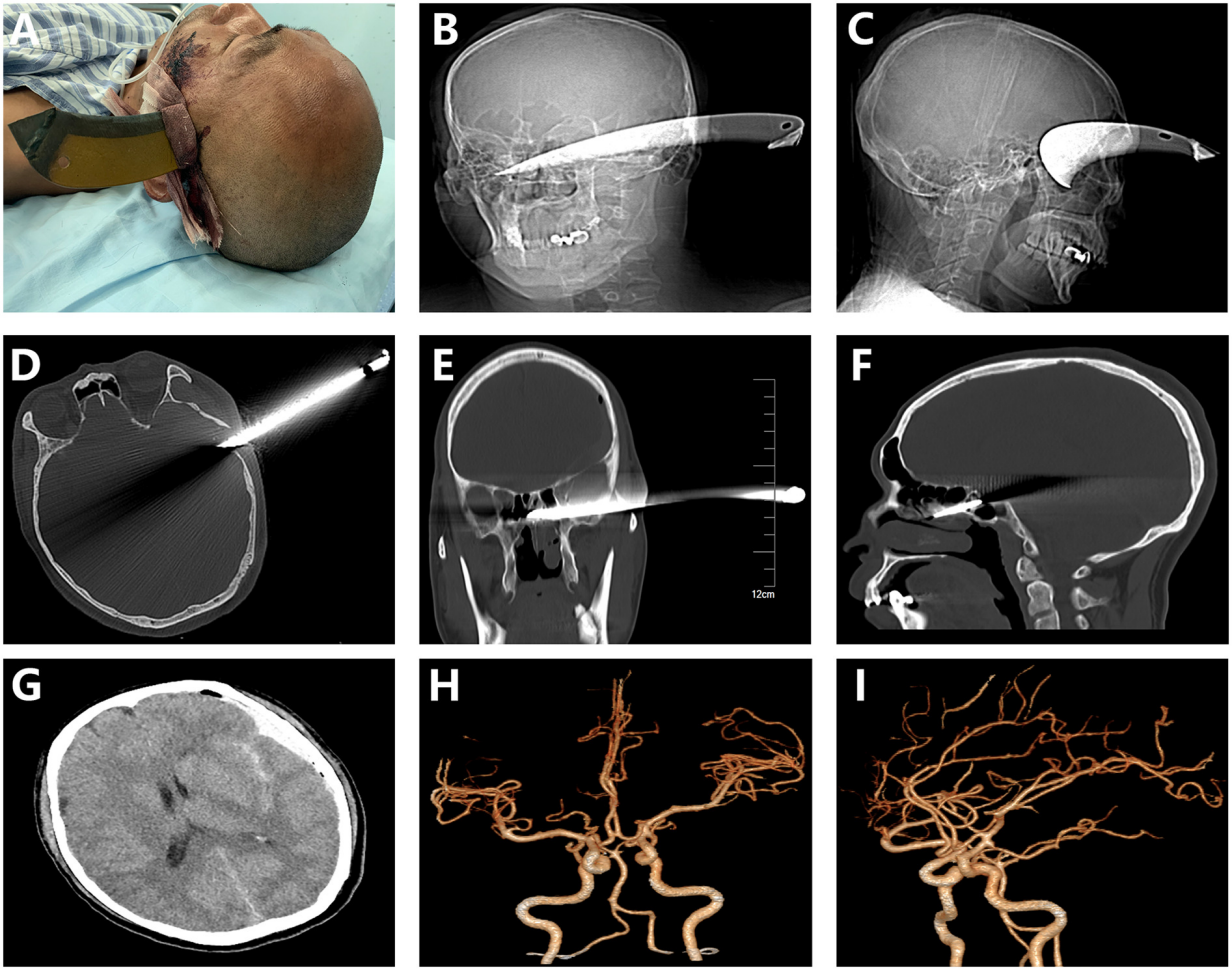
The preoperative image depicted a metallic foreign body that penetrated through the left temporal region (A). Radiographic examination, along with computed tomography (CT) scans, clearly demonstrated that the metallic foreign body traversed the left temporal lobe, passed through the ethmoid and sphenoid sinuses, and extended to the sphenoid bone and the posterior part of the right orbit (B–F). Axial CT imaging revealed the presence of subdural hematoma and subarachnoid hemorrhage (G). Computed tomography angiography (CTA) findings indicated that there was no major injury to the intracranial arteries (H,I).
